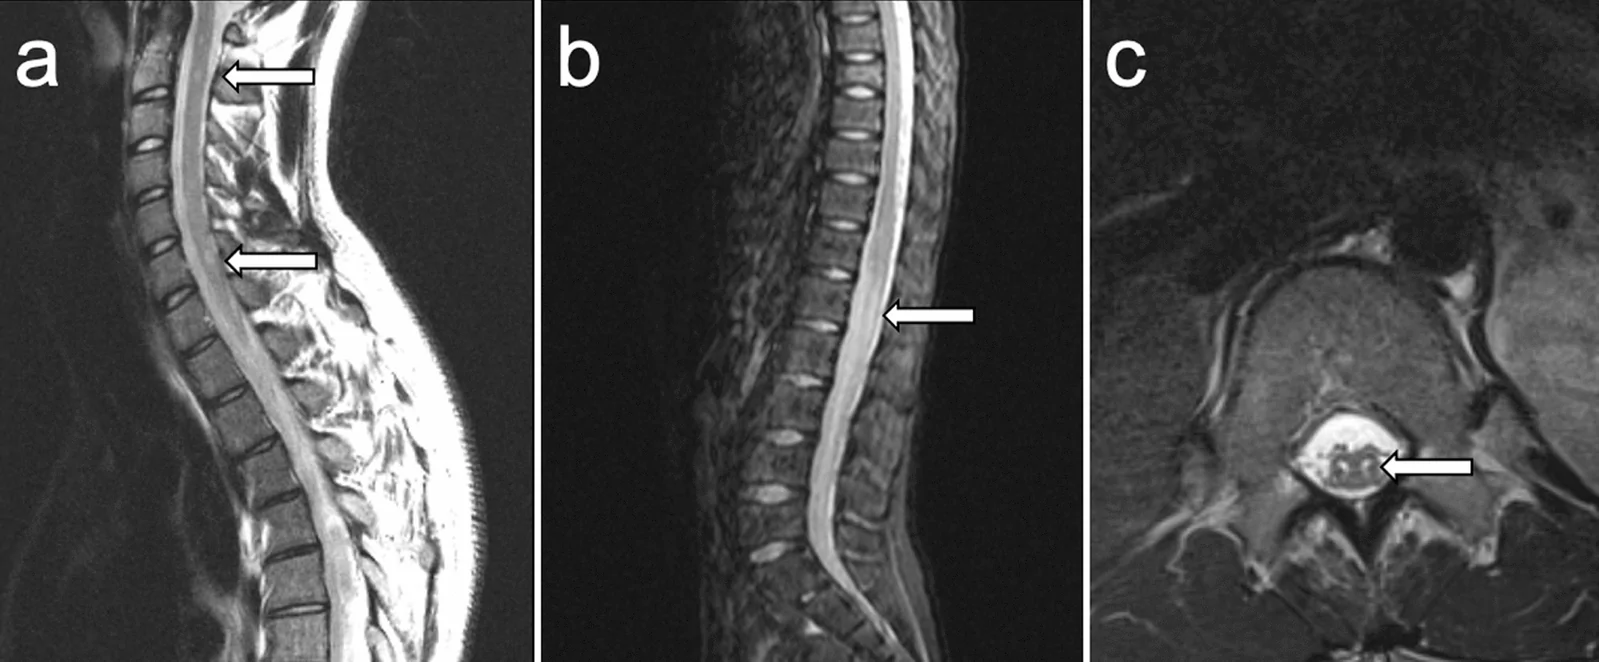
Neuroimaging findings demonstrating spinal lesions in three additional cases. (a) Case #4: Sagittal T2-weighted MRI obtained 1 year after LI, showing a hyperintense lesion at the C5–C6 level. (b) Case #16: Sagittal T2-weighted MRI obtained 18 days after LI, demonstrating contrast enhancement of the cauda equina and nerve roots. (c) Case #28: Axial (transverse) T2-weighted MRI obtained 70 days after LI, showing a symmetrical lesion of the conus medullaris
